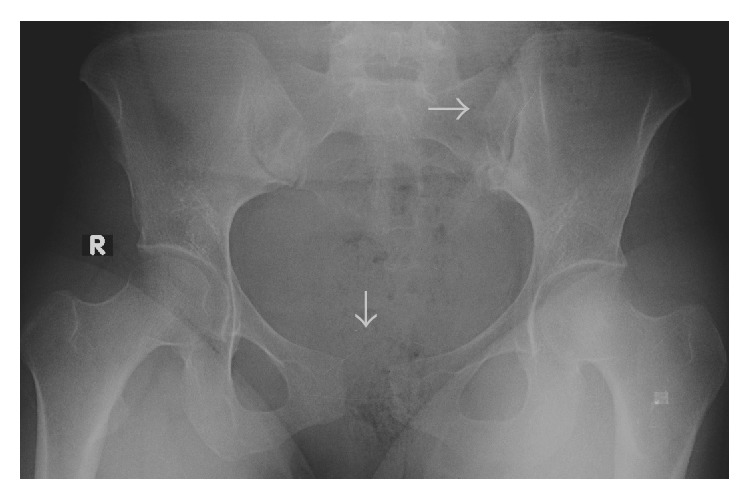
Anteroposterior radiograph of the pelvis demonstrating gross disruption of the pubic symphysis, with a separation of 2.4 cm and disruption of the left sacroiliac joint.
